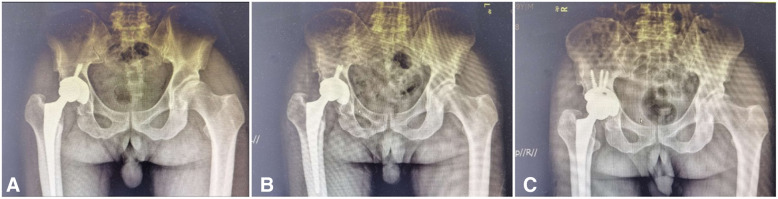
Plain radiographs at 32 months (a) showed initial eccentric wear of the prosthesis. Seven months later, the HXLPE was penetrated and partial inner wall of acetabular shell was worn (b). Plain radiographs of the pelvis showed ideal size and position of the prosthesis (c)
